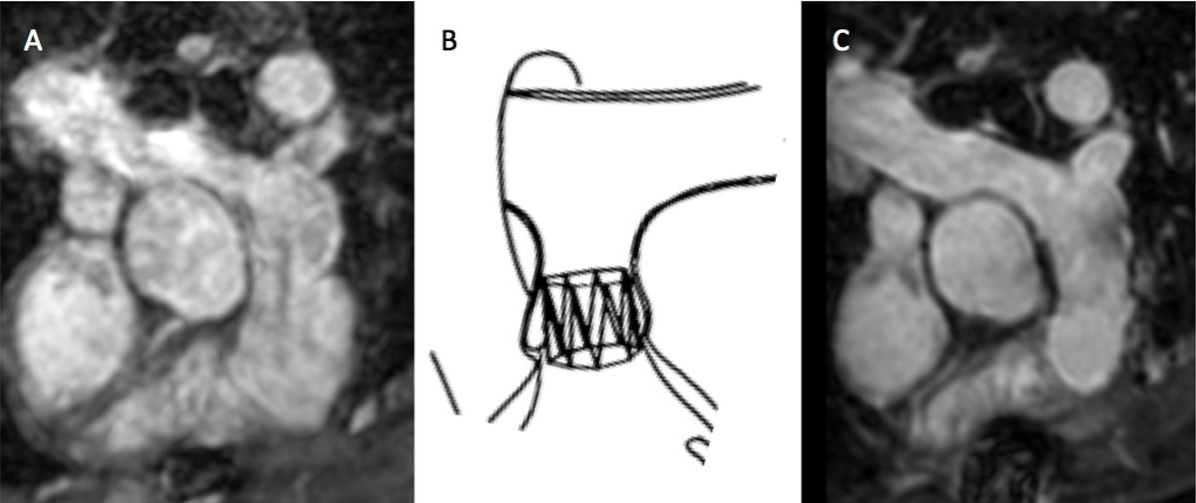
3D Whole-heart imaging of the MPA pre (A) and post (C) IPV implantation shows well seated device according to the draft (B) with low magnetic susceptibility of the device and adequate intra-stent visualization (no need for furter investigation such as CT or catheter).
